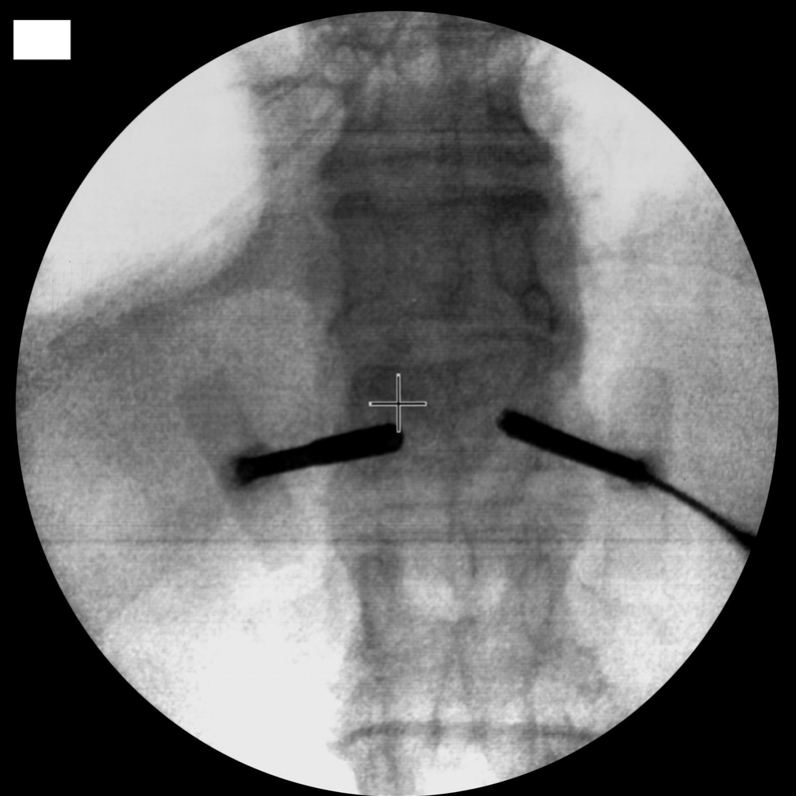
X-ray control of guiding sleeve position.
